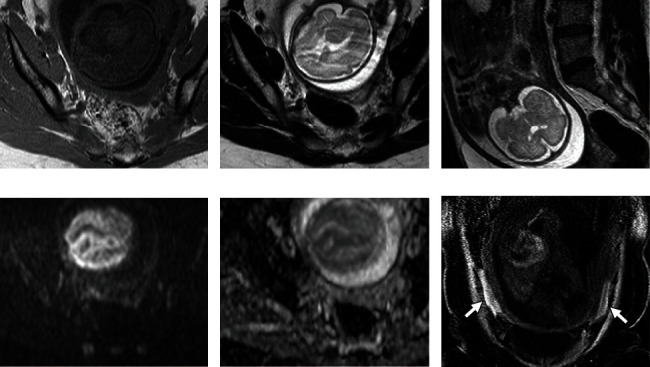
Magnetic resonance imaging (MRI) of the pelvis performed at 27 weeks of gestation. (a) Axial T1-weighted image. (b) Axial T2-weighted image. (c) Sagittal T2-weighted image. (d) Axial diffusion-weighted image (b factor: 1000 s/mm^2^). (e) ADC map. (f) Coronal T1-weighted image with fat saturation. The cystic lesion disappears (a–e). Ascites around the uterus shows high signal intensity on fat-suppressed T1-weighted images, which is consistent with bloody ascites (f, arrows). Based on serial MRI findings, rupture of the decidualized ovarian endometrioma is suspected.
